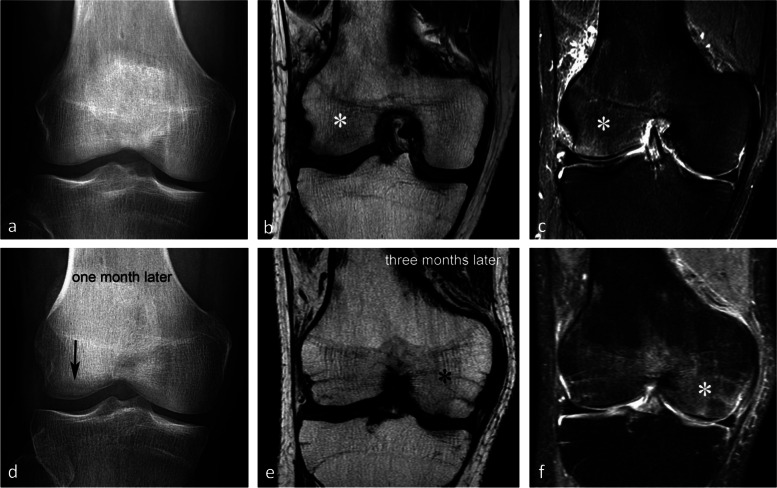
Migrating bone marrow edema (BME)-like lesion in CRPS1. a In early phase, the radiograph (a) was normal. b, c However, MR images showed typical BME-like signal appearing as low and high signal intensity on T1- (b) and fat-suppressed T2-weighted (c) images, respectively, in the lateral condyle (asterisks). d One month after the initial examination, radiograph show bone loss at the same location (arrow). e, f Three months after the initial examination, T1- and fat-suppressed T2-weighted images (e and f) show almost normal lateral condyle, while BME-like signal intensities have appeared in the medial condyle (asterisks)
